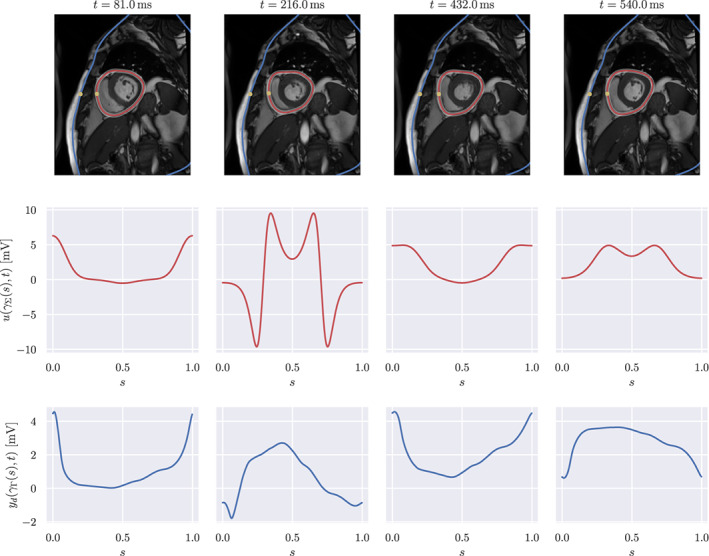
Geometry and input data. First row: cardiac magnetic resonance images with superimposed segmented chest (blue) and time‐dependent pericardium (red). The shaded region around the pericardium represents the shape confidence interval, obtained as $E\left[\sum \right]\left(t\right)\pm 1.96\cdot \text{StdDev}\left[\sum \right]\left(t\right)$. The yellow dots correspond to $\gamma_{\Gamma }\left(0\right)$ and $\gamma_{\sum_{\mathrm{ref}}\left(t\right)}\left(0\right)$. Second row: forward data $u\left(\gamma_{\sum }\left(s\right),t\right)$. Third row: inverse data $y_{d}\left(\gamma_{\Gamma }\left(s\right),t\right)$
